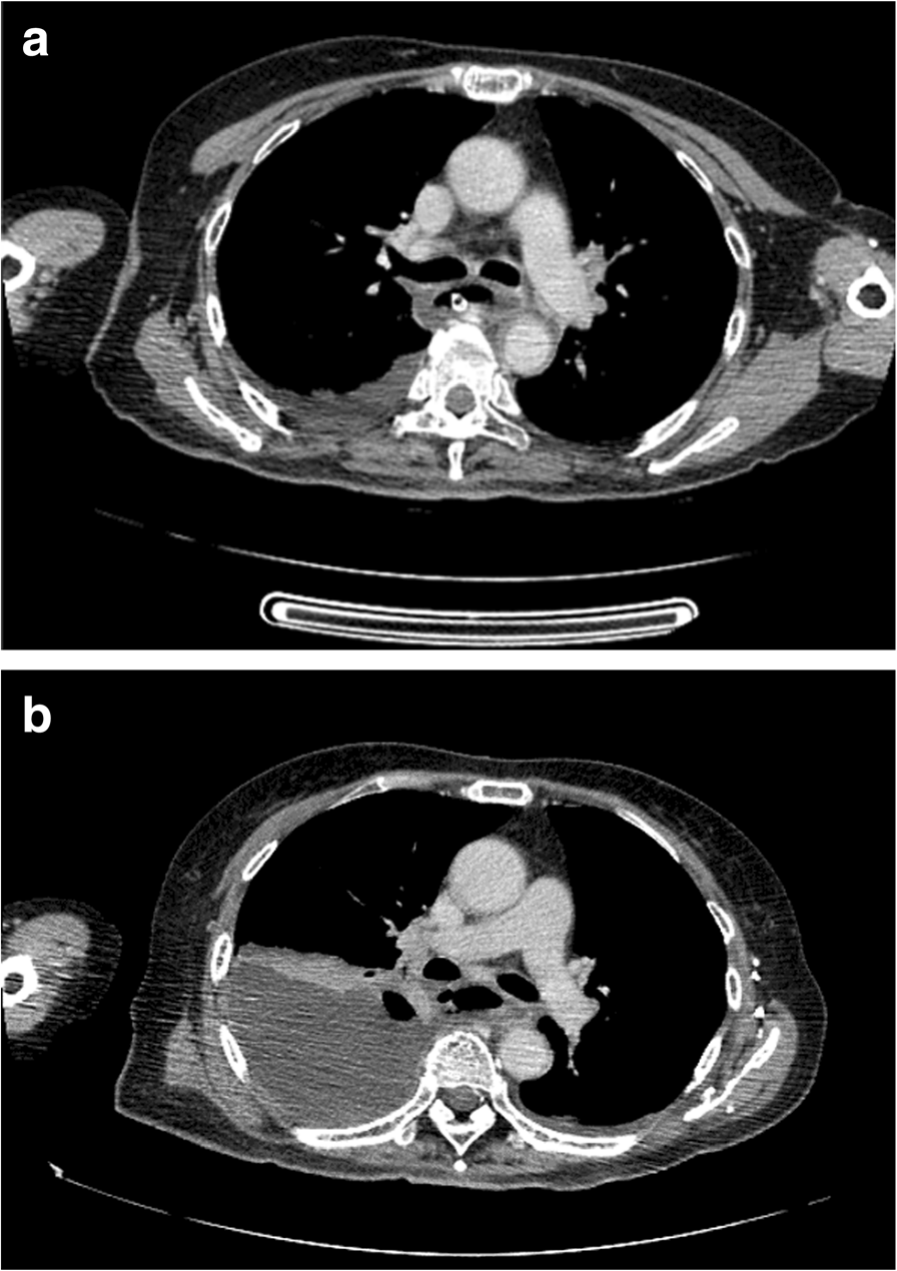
CT scan on admission day and on the 7th day after admission. a Circumferential edematous thickening of the esophageal wall with slight pleural effusion. b CT scan showed a significant increase of the right pleural effusion and free air in the mediastinum
